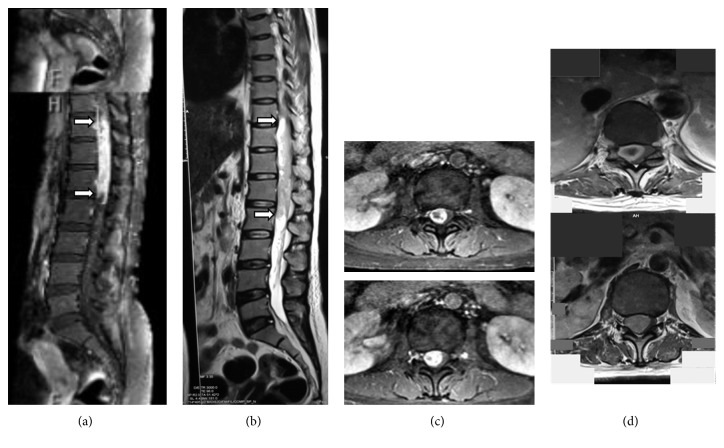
Thoracolumbar T2W1 MRI in the sagittal plane (a and b) and axial plane (c and d) at the tumor height. We can observe the growth of hyperintensity from T10 to L2 in (b) compared with the initial MRI taken (a). Tumor margins are marked with the arrows. Axial T2W1 images on the tumor edges are shown in (c) and (d); the tumor diameter and length have increased in the space of four months.
